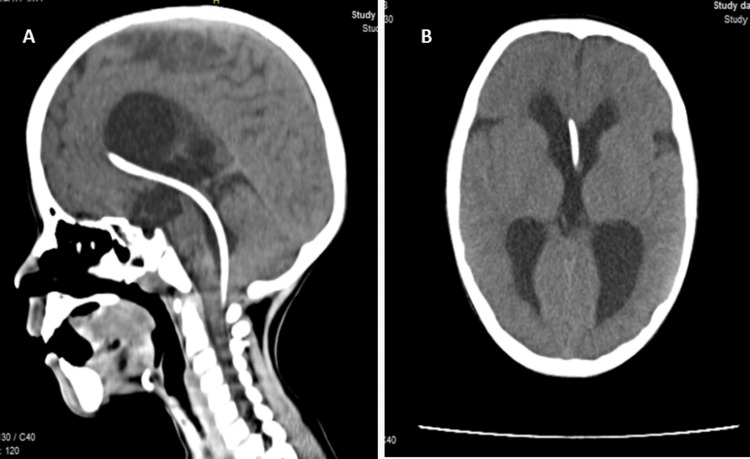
Control CT scan (four weeks after reintervention). A - Sagittal view showing the catheter’s trajectory, proximally from the right lateral ventricle and placed distally into the spinal subarachnoid space; B - Axial view shows the proximal catheter from the right lateral ventricle passing through the foramen of Monro to the third ventricle.
